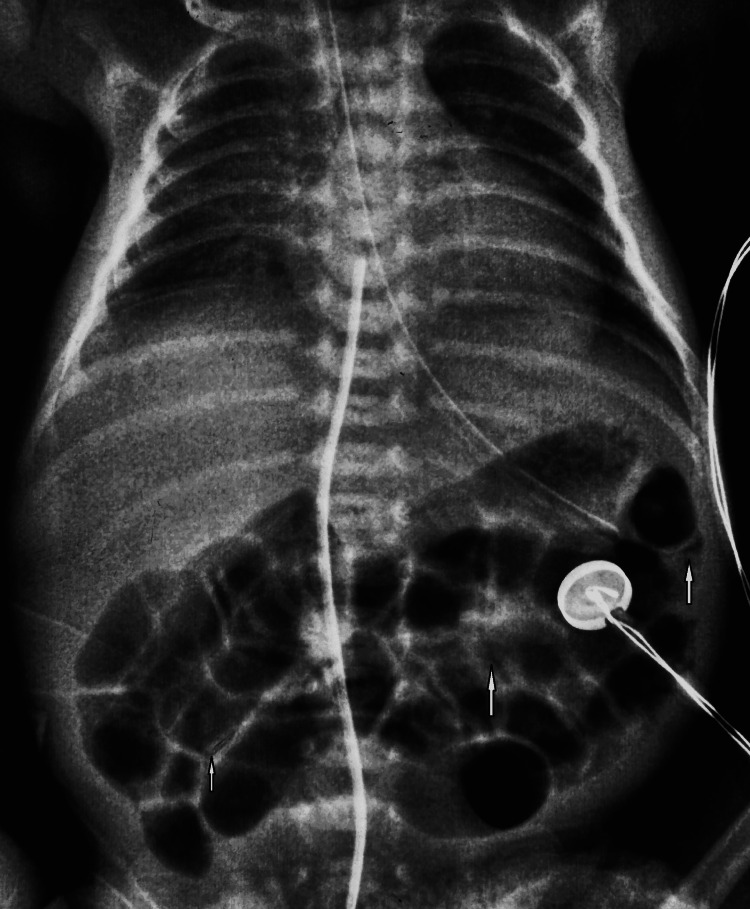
Abdominal radiograph showing intramural gas or pneumatosis intestinalis represented by white arrows, suggestive of NEC IIa.
